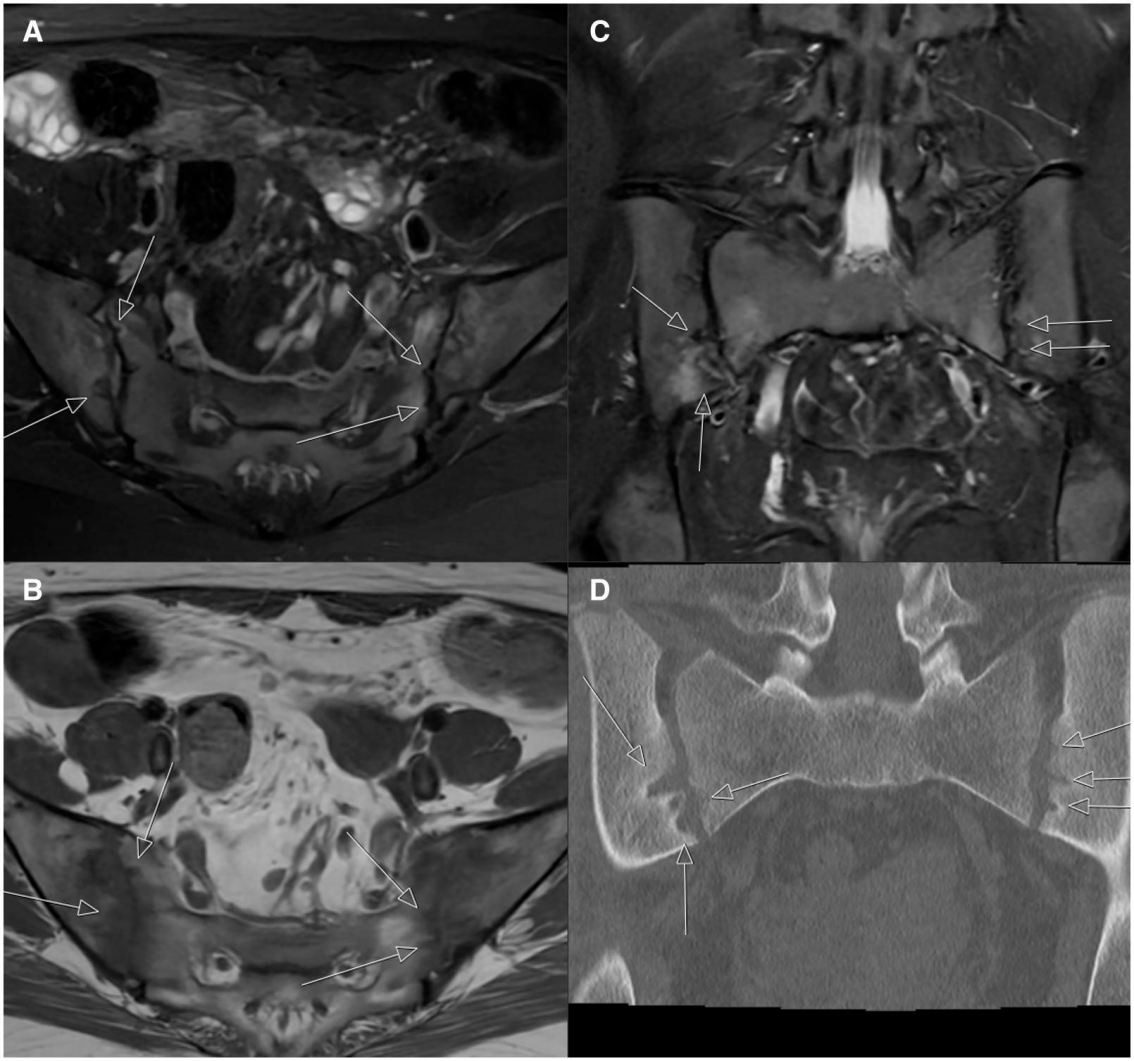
Images of widespread subchondral signal increase compatible with oedema are observed on axial fat-suppressed T2-weighted sacroiliac MR images (A), and signal loss compatible with oedema is observed in the same locations on axial non-contrast T1-weighted sacroiliac MR images (B). In the coronal STIR sequence (C), an increased subchondral signal compatible with oedema was observed. (D) Coronal image of ldCT. White arrows indicate areas of accompanying millimetric erosion. Figure emphasizes that while the erosion is noticeable on MRI, it is more clearly visible on CT.
